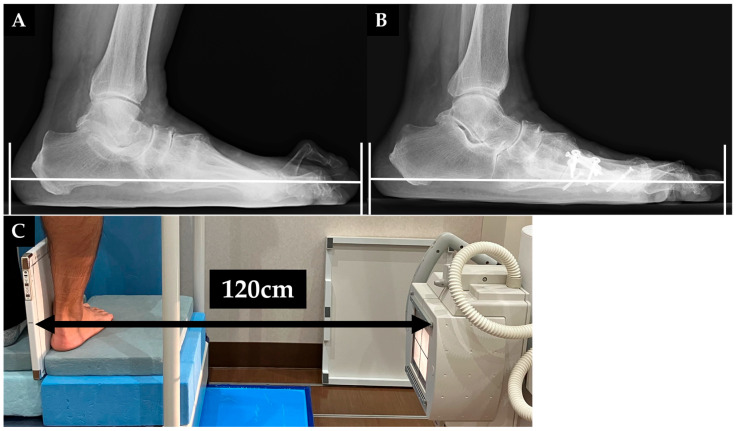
Preoperative (A) and postoperative (B) measurements of radiologically determined foot length on lateral weightbearing radiographs. Radiologically determined foot length was defined as the distance between the shadow of the heel to the tip of toe (white line). The patients stood on the table; then, their feet were positioned in a natural standing posture, ensuring that the toes faced directly forward. The detector was placed vertically against the inner side of the foot. The focal spot-to-film distance was set to 120 cm. The incidence angle was 0 degrees (C).
